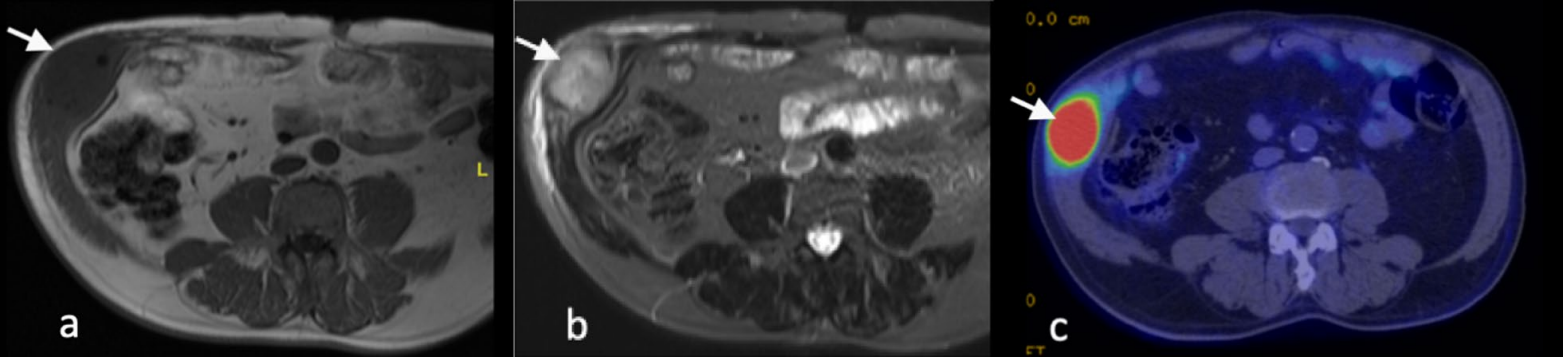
a T1-weighted and (b) T2-weighted fat suppressed MR and (c) PET-CT images of TCC recurrence within the right anterolateral abdominal wall scar (white arrow)
